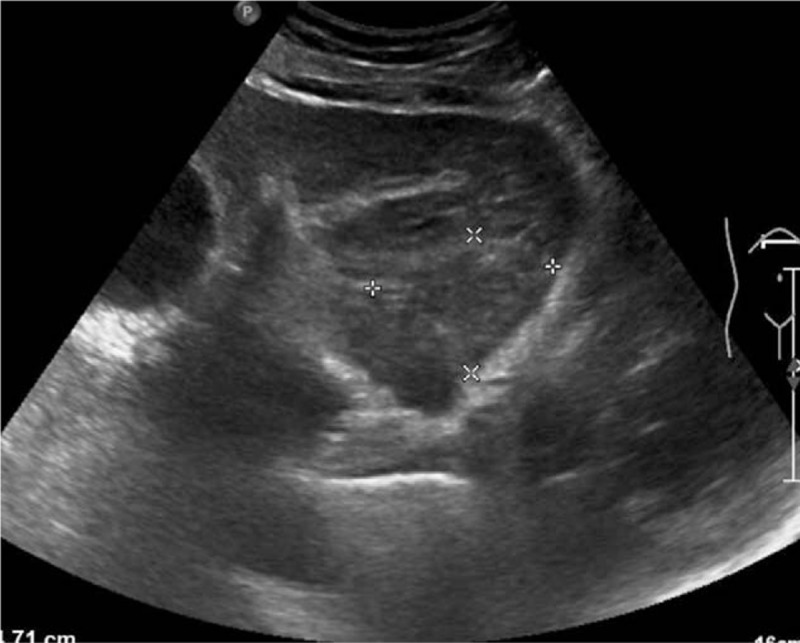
Abdominal ultrasonography. A well-defined, heterogeneously hypo-echoic mass (4.7×3.6 cm in size) was found in the left lateral liver. The boundary and vascular network were not clearly defined.
